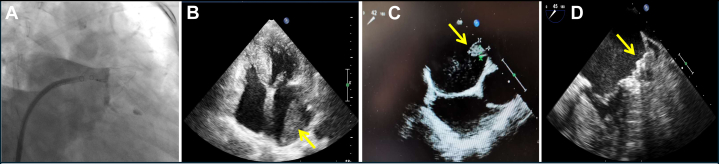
Device-Related Thrombosis Visualization and Removal (A) Successful implantation of a 33-mm Watchman occluder with no detectable peridevice leaks. (B) A left atrial thrombus (5.2 cm × 2.5 cm) was revealed on transthoracic echocardiography (TTE) at the 6-month follow-up. (C) The thrombus (2.1 cm × 1.7 cm) was shown on transesophageal echocardiography (TEE) at the 9-month follow-up (3 months after initiating anticoagulation). (D) Sustained complete thrombus resolution was demonstrated on TEE at the 18-month follow-up (12 months after initiating anticoagulation).
